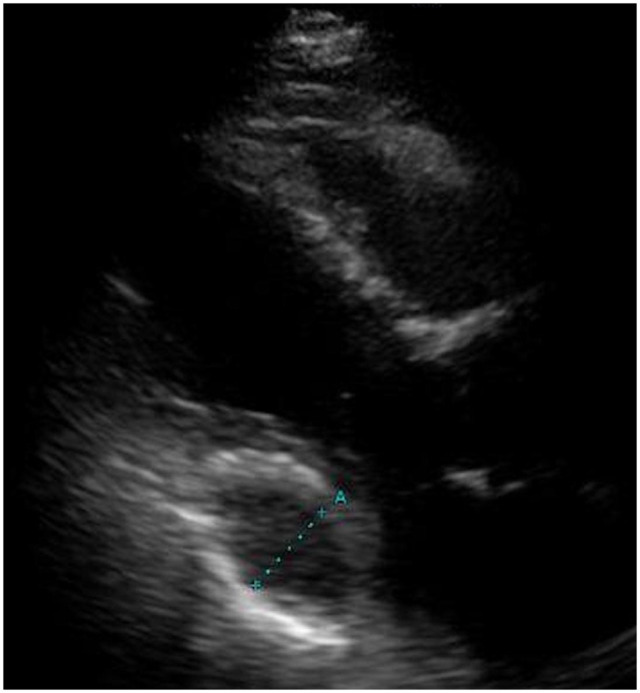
On transthoracic echocardiography, the mitral leaflets appeared compressed by the pericardial mass located posterior to the left ventricle and deviated anteriorly.
